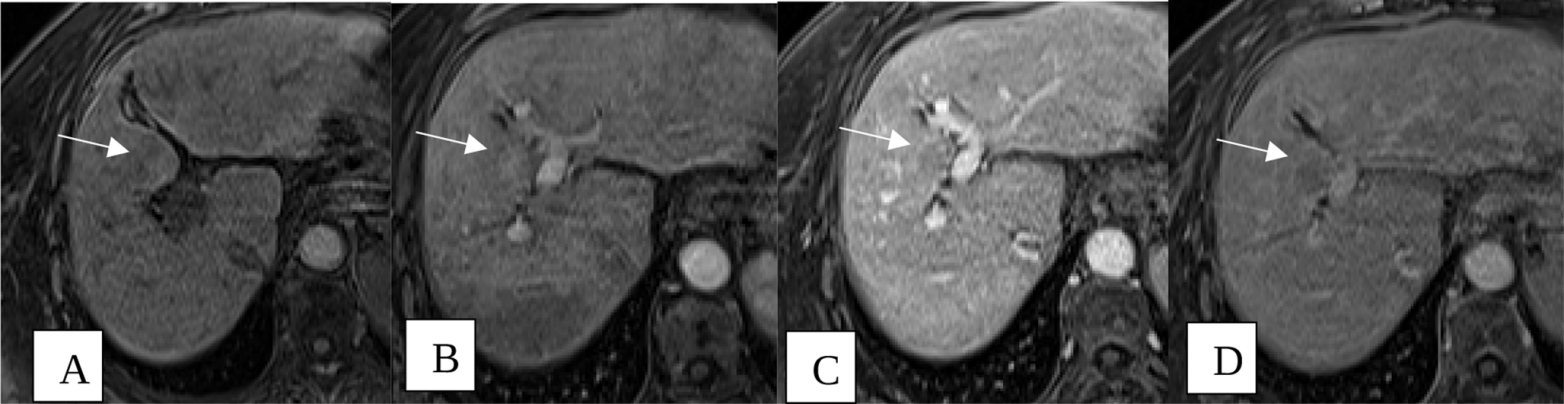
57-year-old male with cirrhosis status post TACE of 1.5 cm segment V/VIII LR-5 observation. Multiphasic MRI of the abdomen with pre-contrast T1 weighted image (A) demonstrating an ill-defined treatment cavity in segment V/VIII. After IV gadolinium contrast administration, there is heterogeneous enhancement in the arterial phase (B), with progressive washout on portal venous (C), and delayed 5-minute (D) T1 weighted images, consistent with LR-TR Viable
